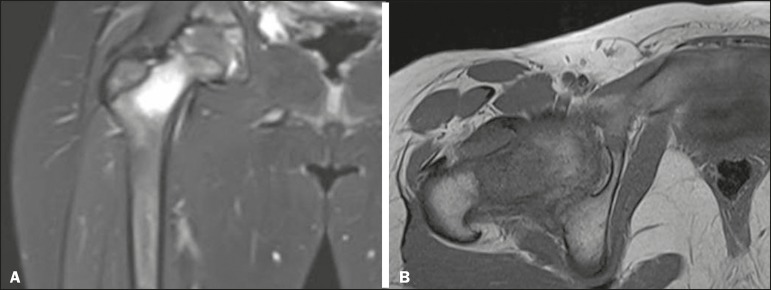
Osteomyelitis in a 9-year-old female. MRI of the right thigh. Coronal fat-suppressed T2-weighted sequence (A) and axial T1-weighted sequence (B) identifying an area in the marrow of the right femoral neck and extending to adjacent muscle planes, with a hypointense signal on the T1-weighted sequence, a hyperintense signal on the T2-weighted sequence, a mild periosteal reaction, and no erosion of cortical bone.
